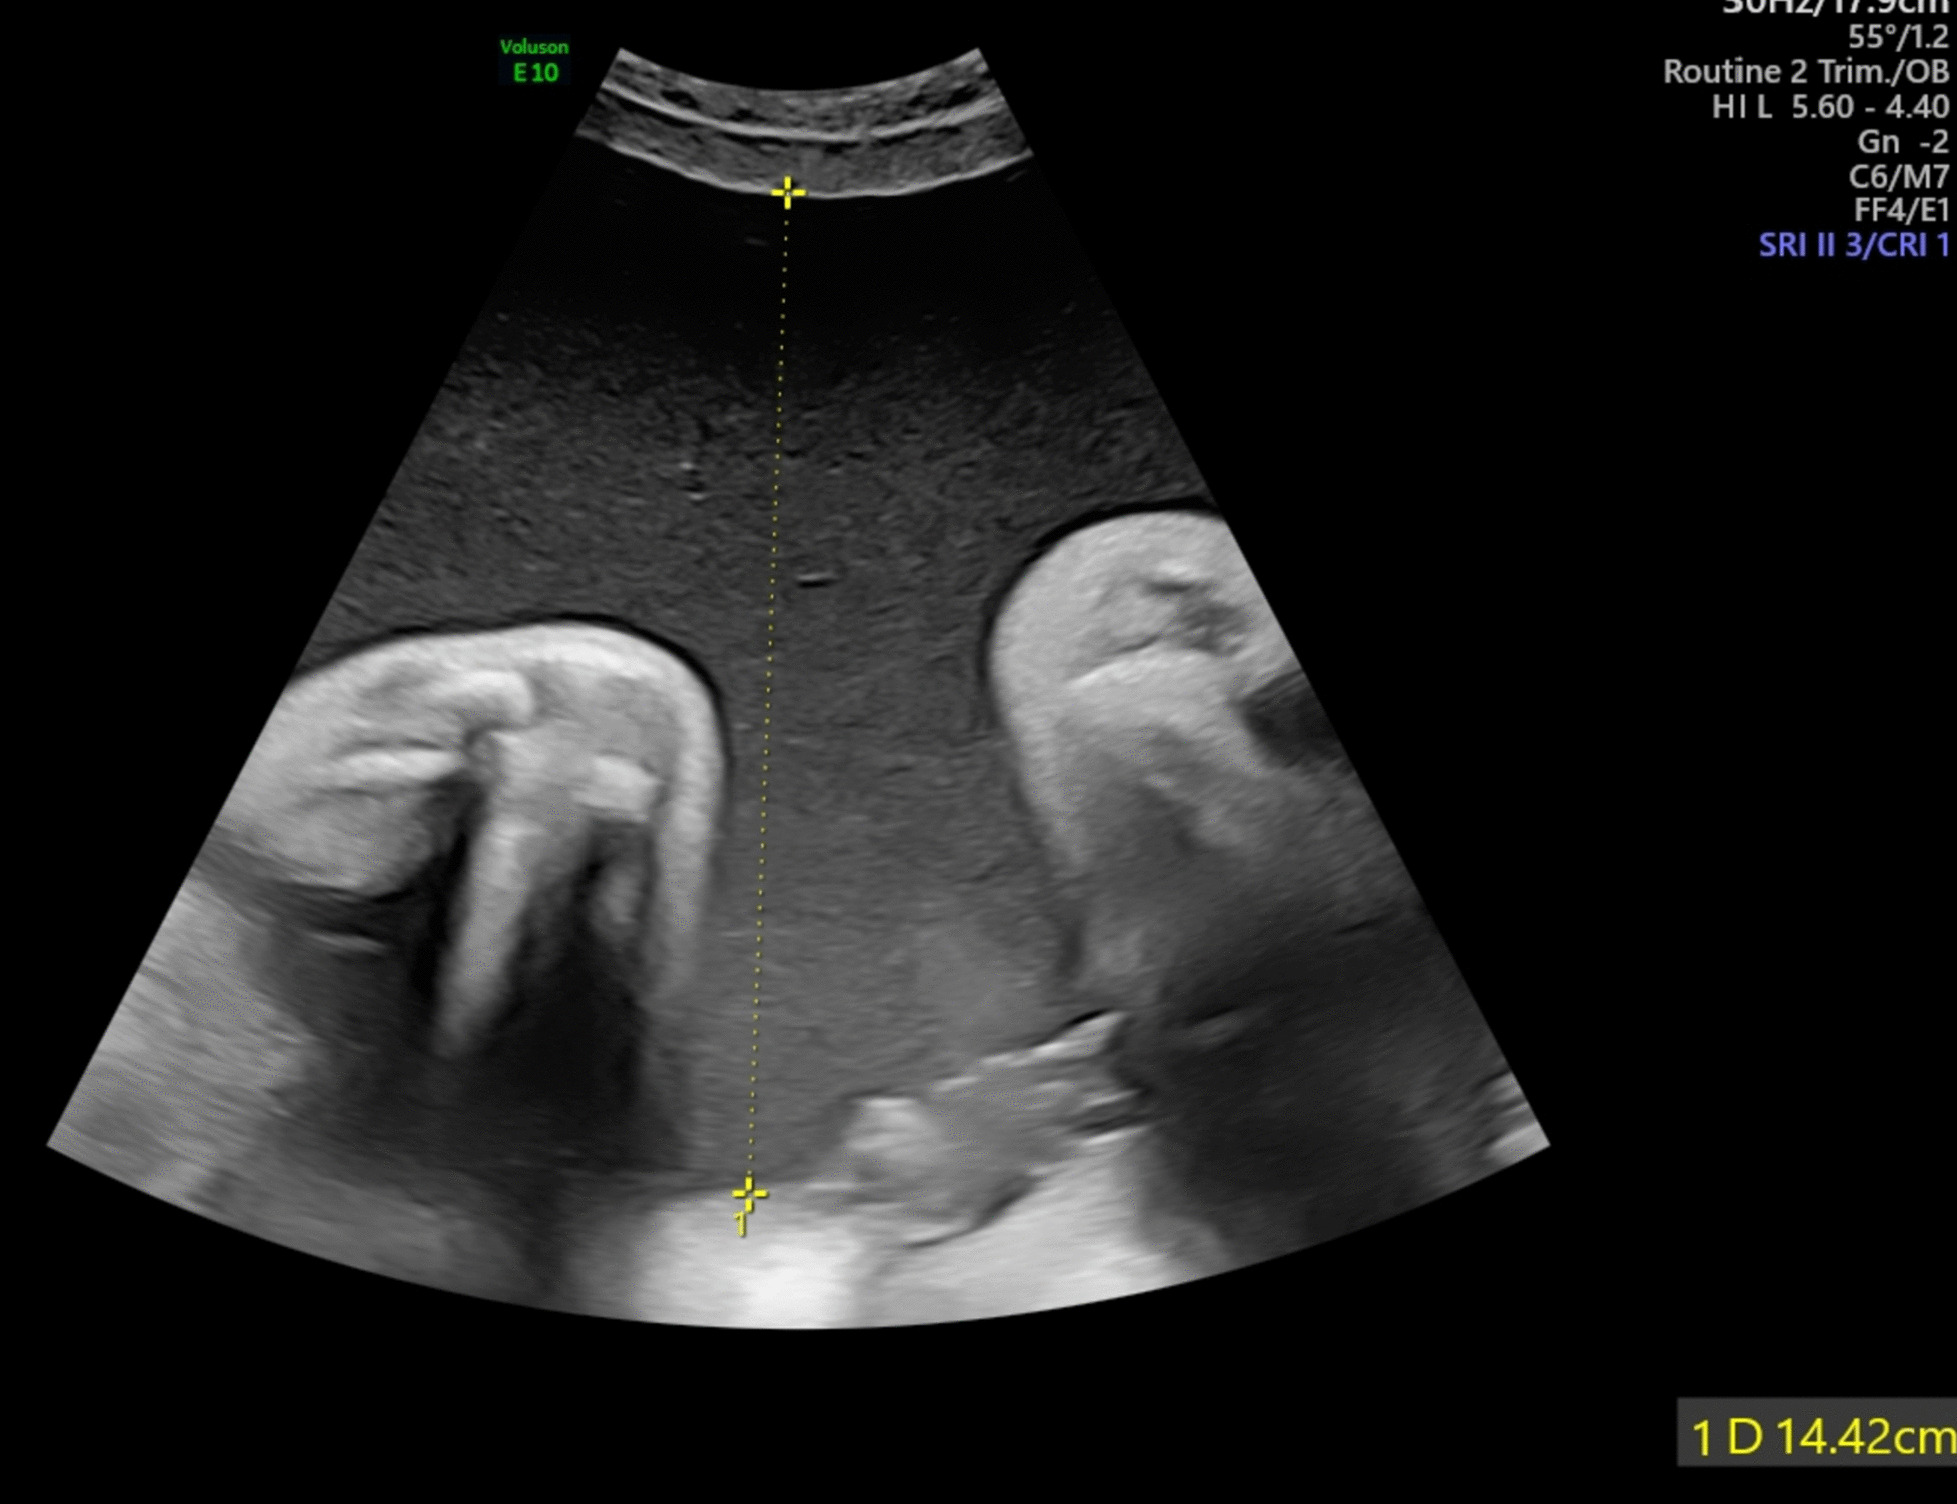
Ultrasound at 31 weeks showing deepest vertical pocket of 14 cm
